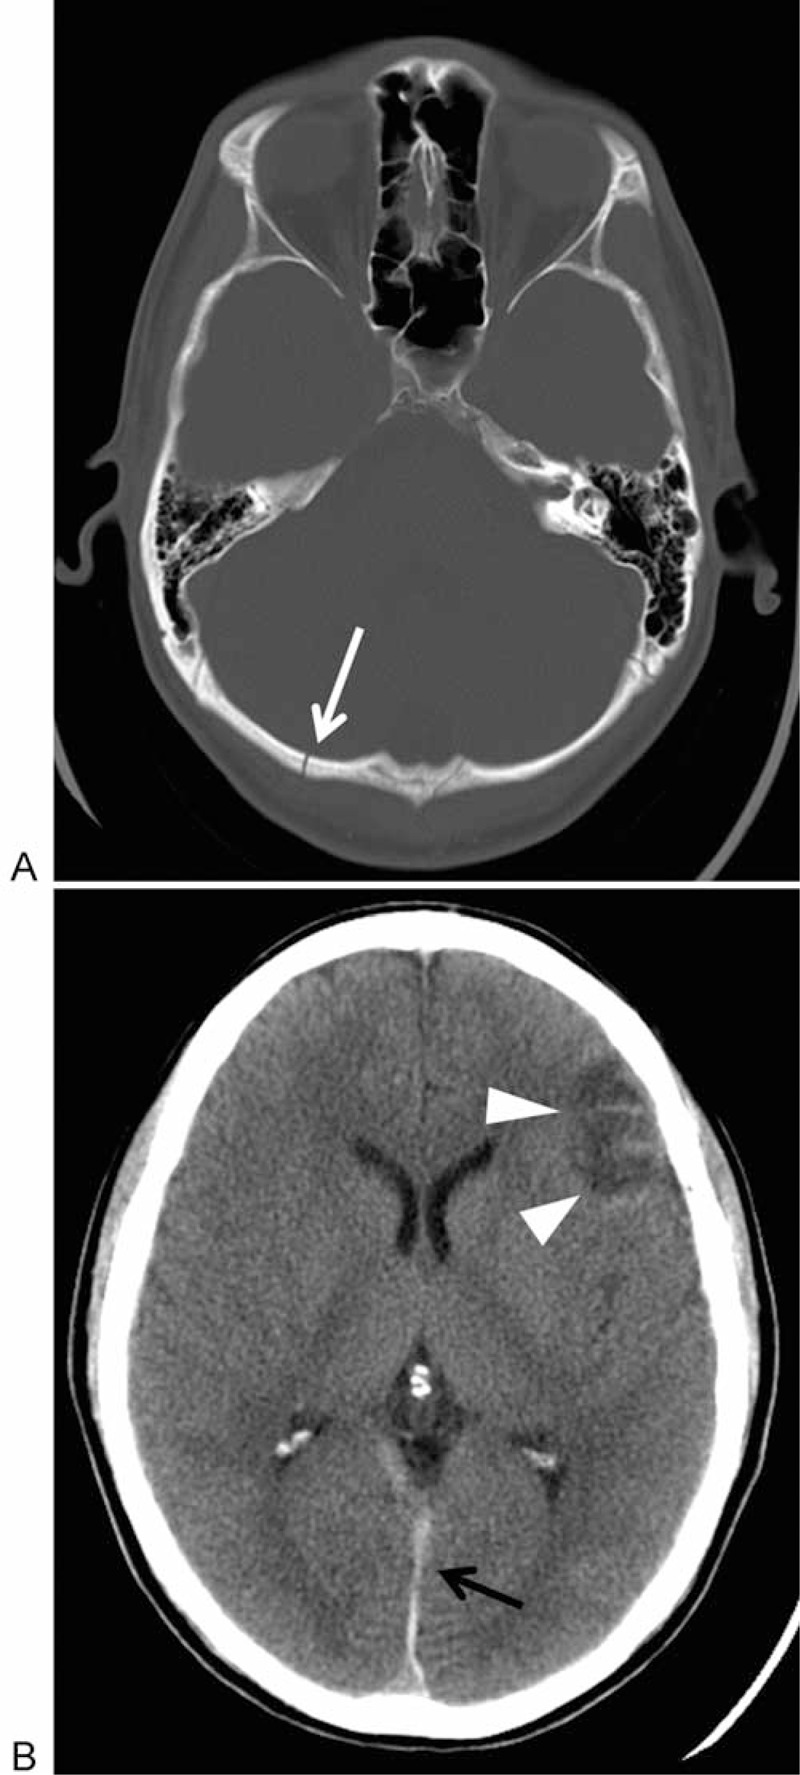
CT of the brain in a 23-year-old male with occipital bone fractures. He fell after alcohol intoxication. Four days later, he visited our ED with the chief complaint of headache and nausea. Axial CT shows fractures of the occipital bone (A, white arrow) and intracranial hemorrhage (B, black arrow) with brain contusion (arrow head).
